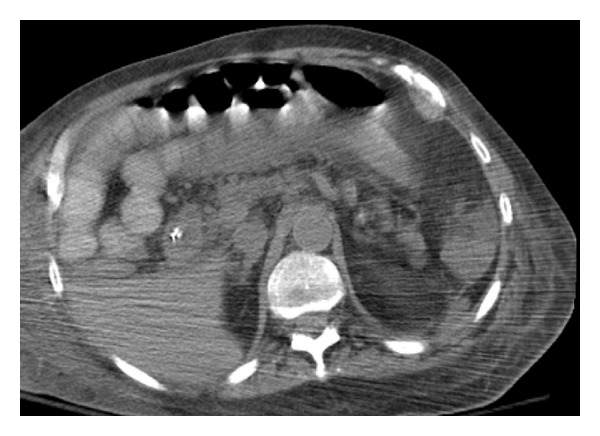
Transverse section on a computerized tomography (CT) scan showing significant relief of the severe impaction and ileus.
